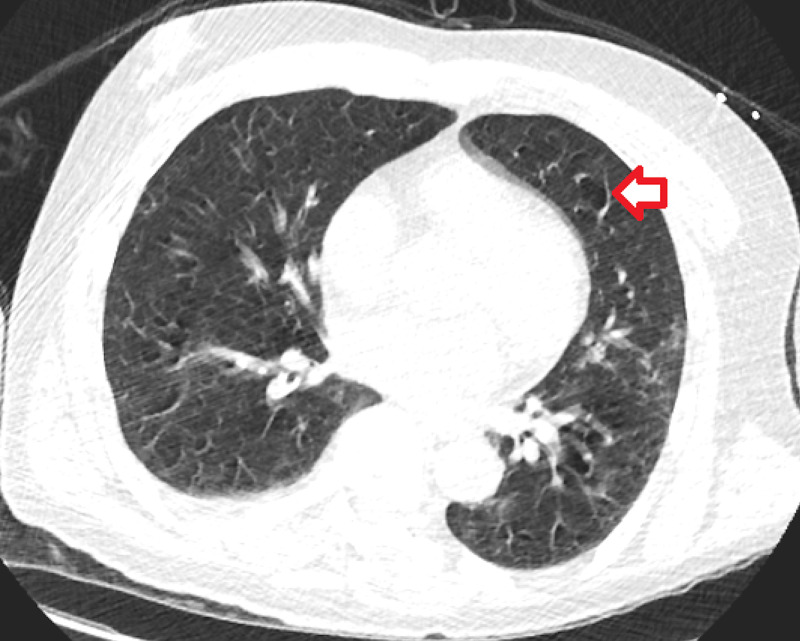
Computed Tomography of the Chest Without Contrast (axial view) Leftwards facing red arrow shows the emphysematous changes.
